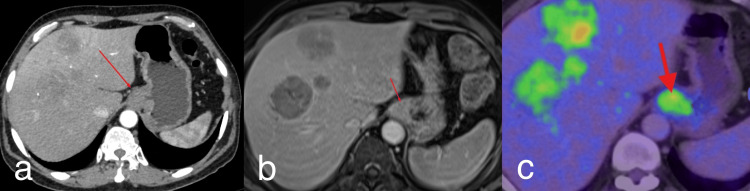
Circumferential enhancing wall thickening. Circumferential enhancing wall thickening at the gastroesophageal junction is seen on contrast-enhanced CT (a) and contrast-enhanced MRI (b), along with fluorodeoxyglucose avidity (red arrow) in PET-CT (c).
